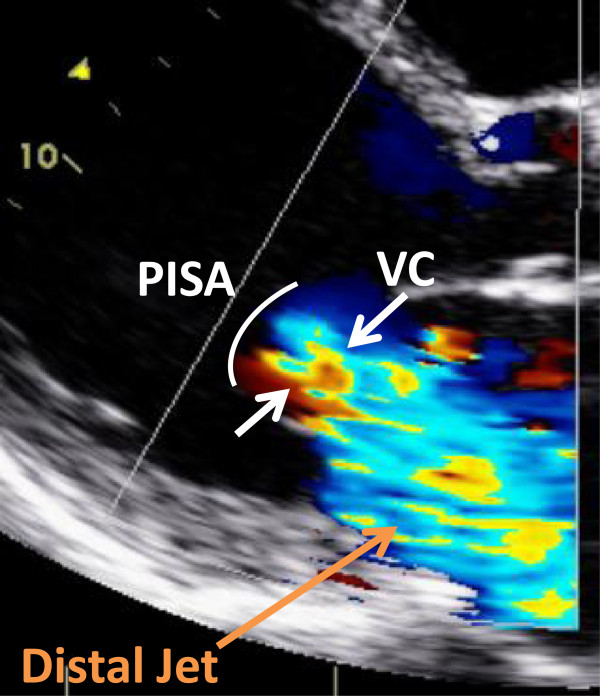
Vena contracta measurement. The VC (white arrows) of the MR jet is measured from parasternal long axis view as the narrowest width of the proximal jet at the level of or just distal to the leaflet tips. In this view, the image is zoomed into the area of the VC (arrows), with sector size and depth selected to optimize color Doppler resolution. Magnification is essential to correct grading of MR severity by the VC method because small changes in the measurement may affect severity grade. VC = vena contracta.
